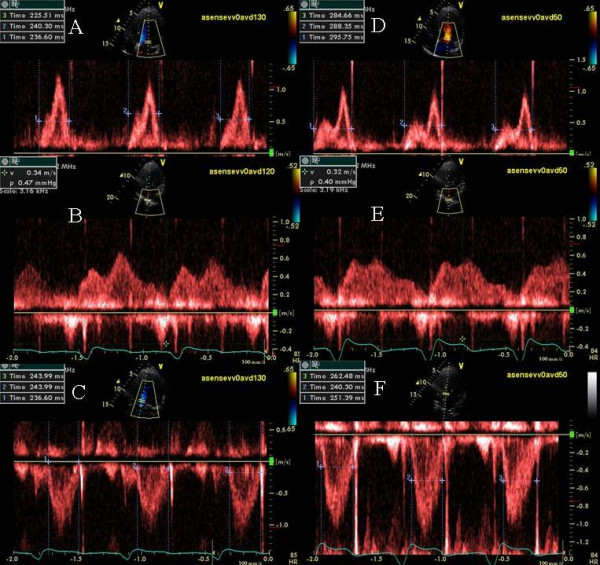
Effect of shortening of AVD on diastolic filling. A, B and C are mitral inflow, pulmonary vein inflow and aortic outflow PW Doppler tracings respectively obtained at an AVD of 130 ms and D, E and F are corresponding PW Doppler tracings obtained at an AVD of 50 ms. Note marked improvement in mitral inflow filling time (from 234 ms to 289 ms), change in pulmonary vein flow from diastolic dominant to systolic dominant along with decrease in atrial reversal velocity (from 34 to 32 cm/s) and improved LV ejection duration (from 242 to 251 ms).
